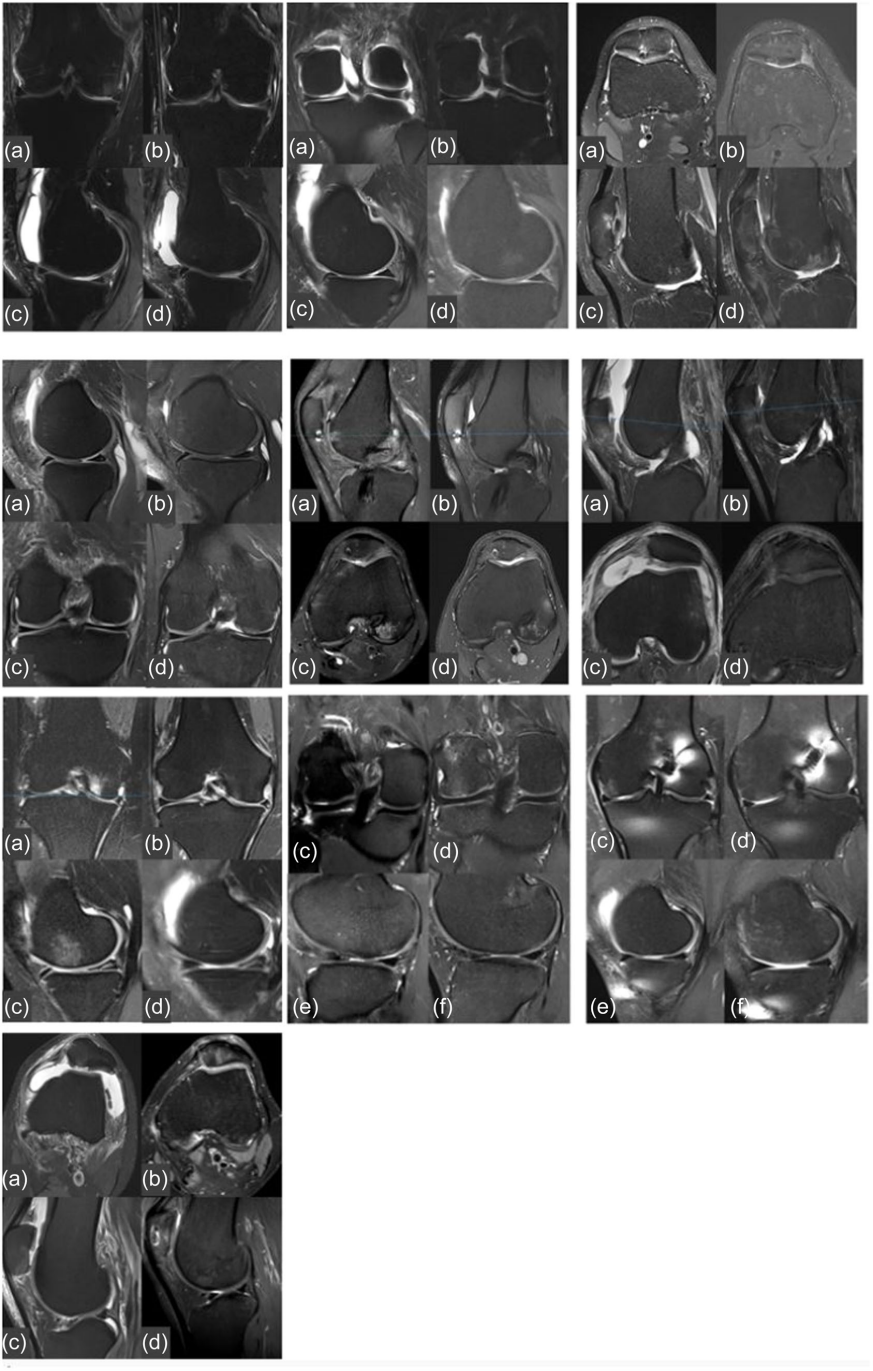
MRI of all patients before surgery (a, c) and 24 months after surgical treatment (b, d). MRI, magnetic resonance imaging.
